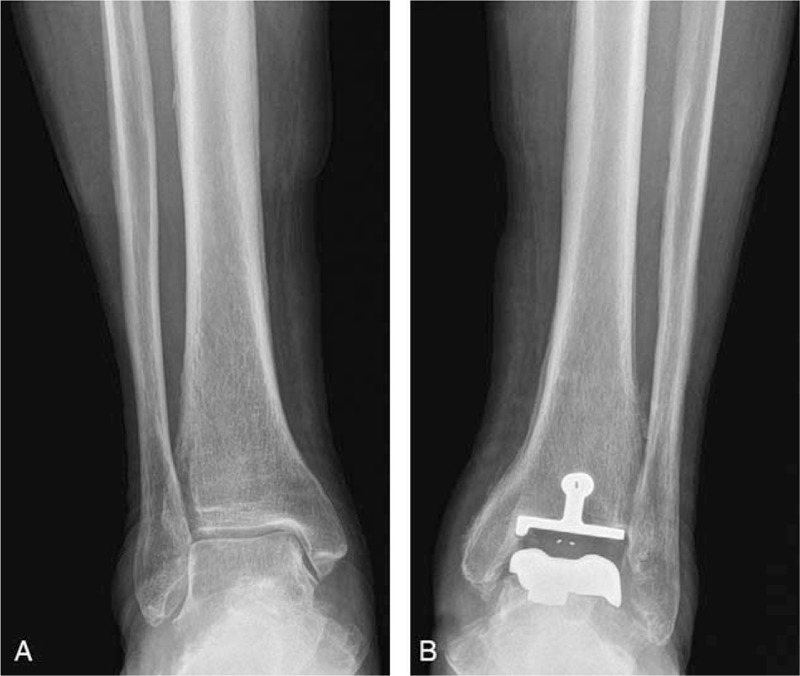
Follow-up frontal radiographs of the right (A) and left (B) lower legs taken 7 months later show thick single lamellar or solid periosteal reactions in both tibias and in the left fibula.
